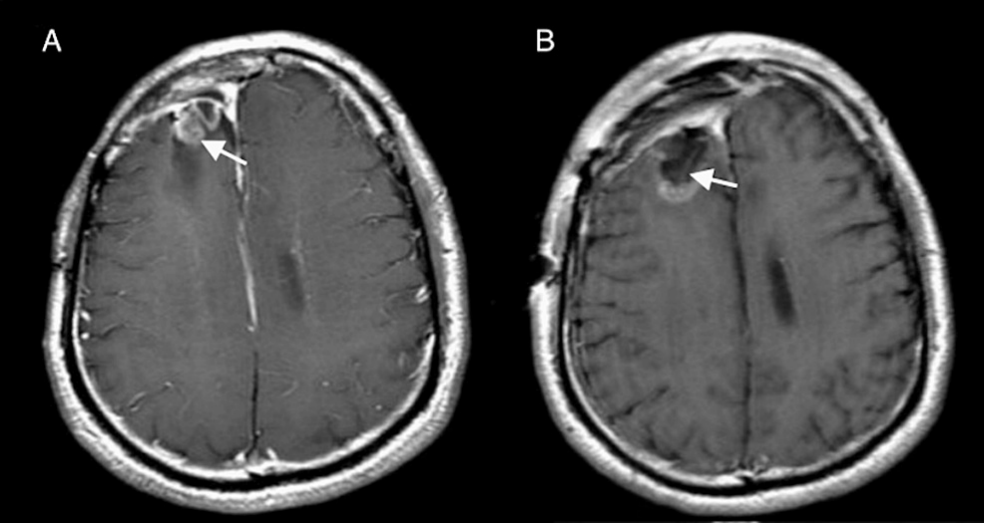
Three-year follow-up: recurrent right frontal tumor A: Preoperative axial postcontrast T1-weighted MRI, three years after initial surgery, demonstrates a 6 mm enhancing nodular focus above the initial resection cavity in the right frontal lobe suggestive of tumor recurrence. B: Postoperative MRI presents gross total resection of the enhancing nodule.
